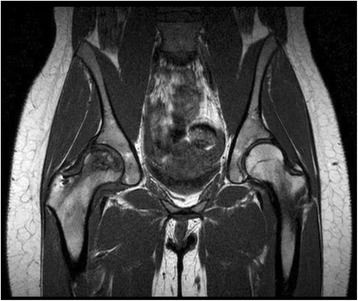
This coronal T1-weighted image shows progression of AVN with necrosis, flattening of the right femoral head, and post decompression evidence in the femoral neck. There is also edema, which corresponds to the increasing pain the patient was experiencing at this time. There are obvious differences between the left and right femoral heads
